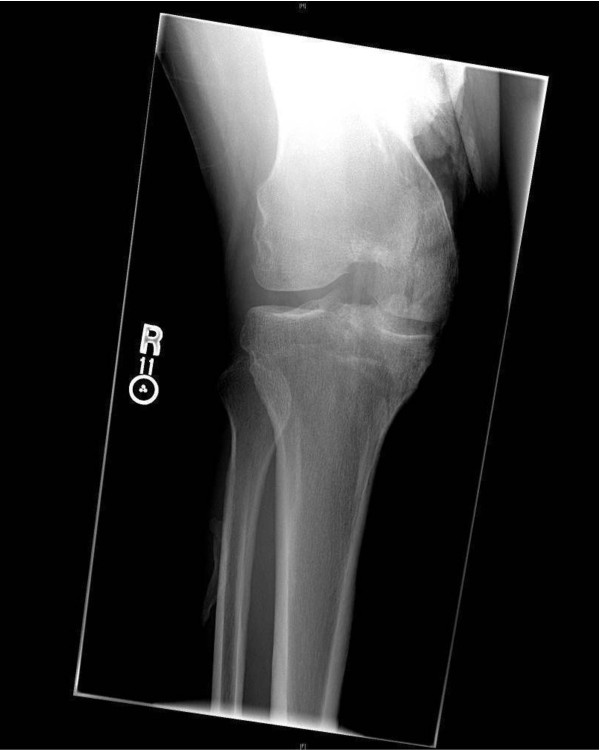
Anteroposterior X-ray of the patient's right knee at the time of presentation. Some HO is seen superior to his knee.
